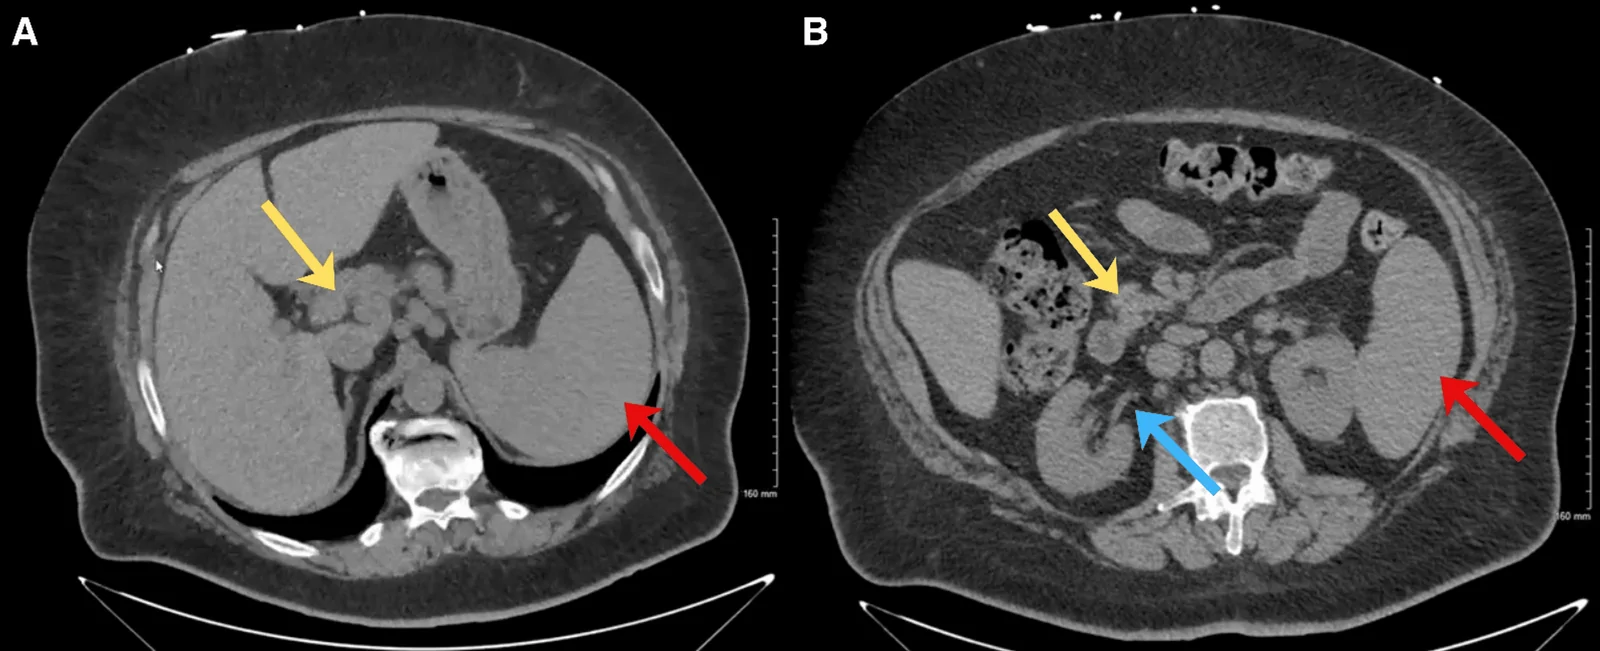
Computed tomography of the abdomen and pelvis demonstrating systemic lymphadenopathy, splenomegaly, and mild hydronephrosis A: splenomegaly (red arrow) and upper abdominal lymphadenopathy (yellow arrow); B: splenomegaly (red arrow), retroperitoneal lymphadenopathy (yellow arrow), and mild right hydronephrosis (blue arrow).
